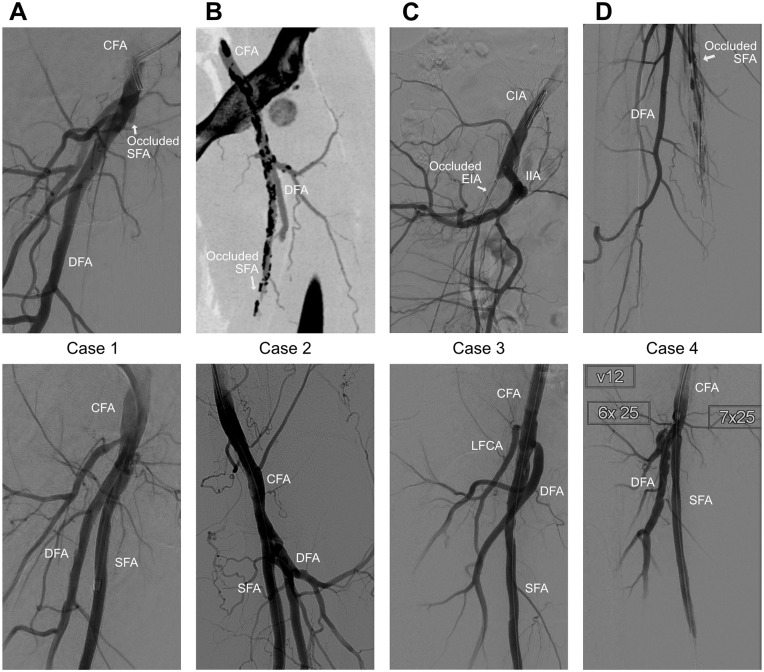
Digital subtraction angiography (DSA) images before (top) and after (bottom) stent graft placement in the SFA. For case 2, a preprocedural CTA-slice is shown, as a DSA before the stent placement was not available. Similarly for case 3, a DSA of the occluded SFA was not available, so a DSA of the occluded external iliac artery is instead shown. CFA, common femoral artery; CIA, common iliac artery; DFA, deep femoral artery; EIA, external iliac artery; IIA, internal iliac artery; LFCA, lateral femoral circumflex artery; SFA, superficial femoral artery.
